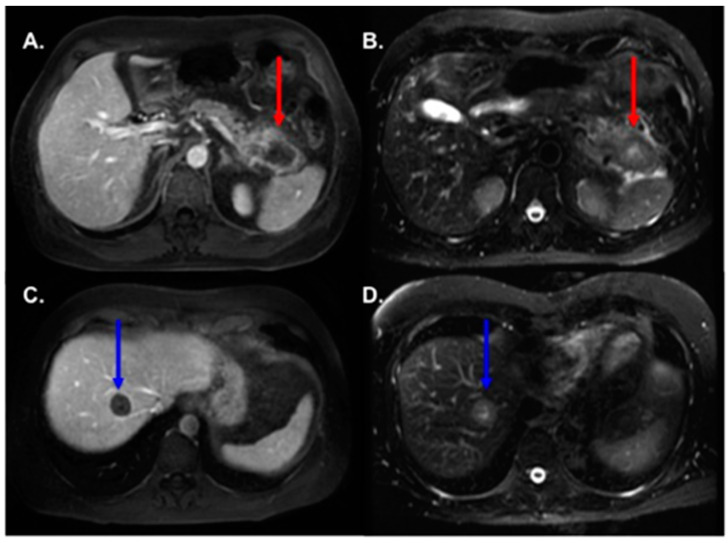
A 51-year-old male with a necrotic mass in the tail of the pancreas. (A) Axial T1 fat-suppressed portal venous phase image demonstrates central hypo-enhancement (red arrow). (B) Axial T2 fat-suppressed image shows associated hyperintensity (red arrow). (C,D) A hypo-enhancing, T2 hyperintense lesion is seen within the liver (blue arrows). Endoscopic ultrasound biopsy revealed primary PDAC with necrosis.
